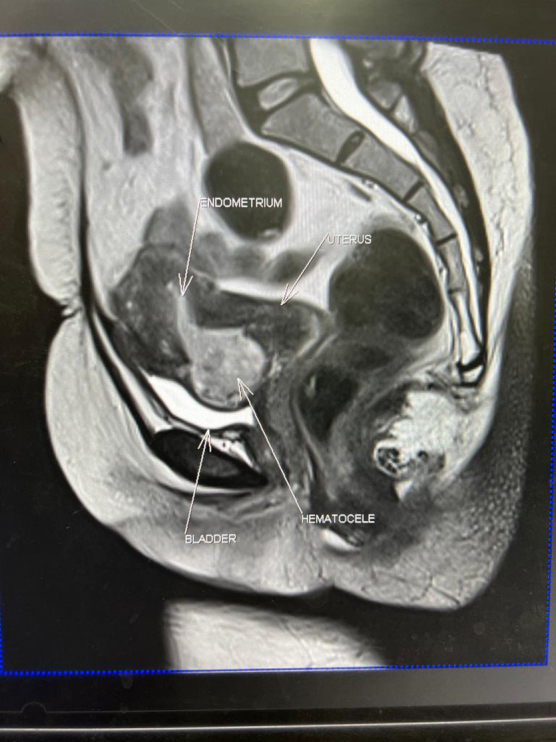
MRI image showing haematocele in caesarean scar site connecting to endometrial cavity. MRI, magnetic resonance imaging.
